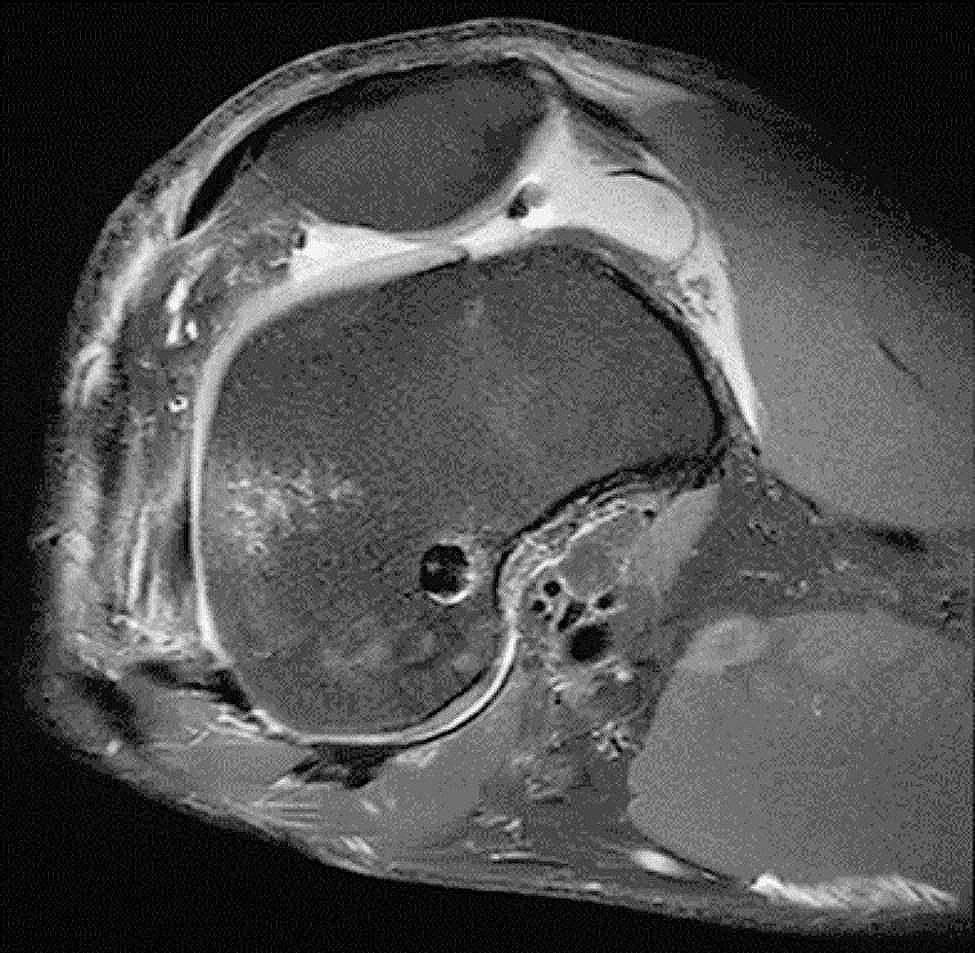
This is measurement of the internal diameter of the femoral tunnel at the level of an MRI scan perpendicular to the femoral tunnel(5 mm from the internal portion of the tunnel)
